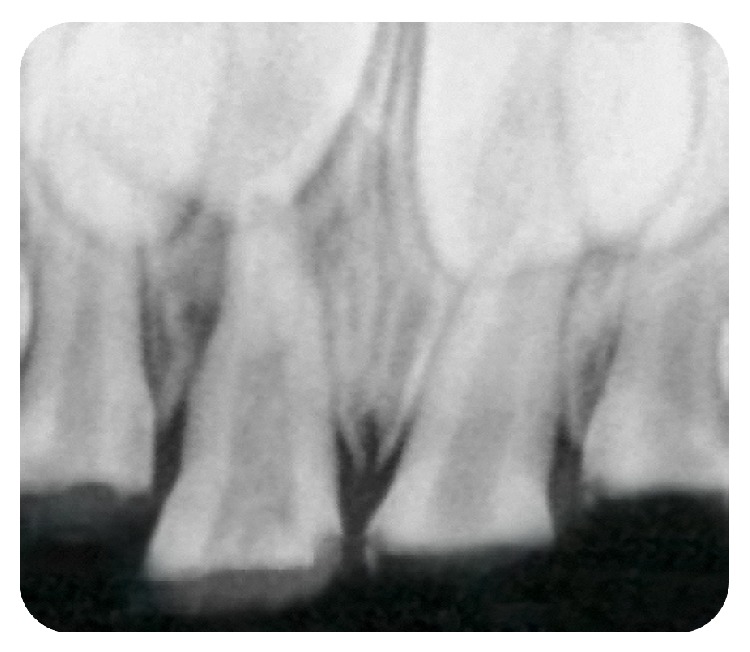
Preoperative radiograph showing teeth 51, 52, 61, and 62.
